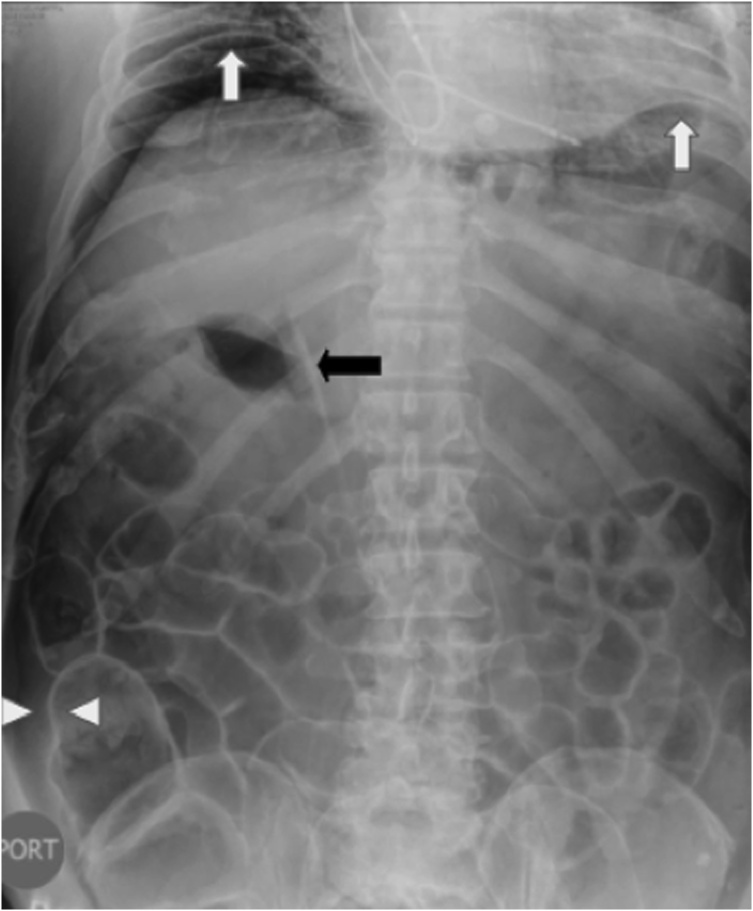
Abdominal radiography showing sub-diaphragmatic free air (white arrows), Rigler´s sign (white triangles), air in both sides of the intestine wall; and Falciform ligament sign (black arrow).
